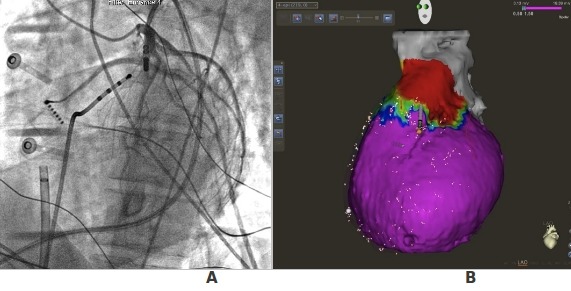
A: Coronary angiography performed with the ablating catheter in a site where, during VT, a diastolic potential was registered. The tip of the catheter is close to the LAD artery. B: Carto bipolar map merged with the epicardial anatomy obtained with a CT. Catheter tip (arrow) is shown in the same position of that registered during angiography.
